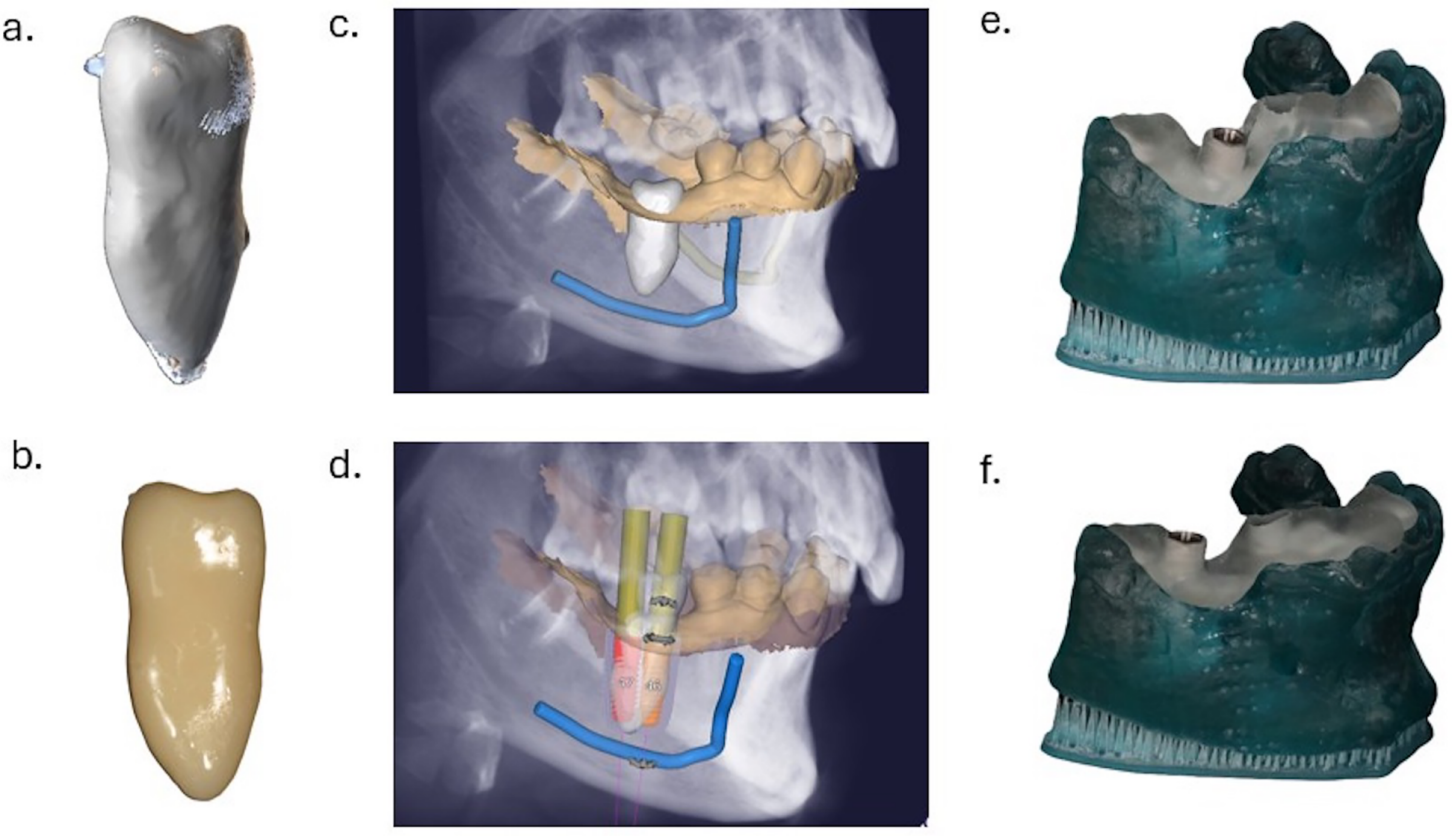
Digital planning for the autotransplantation procedure: digital segmentation of the donor tooth and the fabrication of a three-dimensional printed donor tooth replica; the digital file of the donor tooth was later placed digitally in the recipient site and two surgical guides following each of the donor tooth's roots were designed and printed. (a) Donor tooth segmentation from the CBCT file using BlueSkyPlan software. (b) 3D printed donor tooth replica. (c) Digital planning of the donor tooth position in the mandibular arch using Exocad software. (d) Planning osteotomy with two surgical guides for the neoalveolus. Since the donor tooth displayed two fused roots, two separate surgical guides were designed for each root. (e) Surgical guide for anterior root. (f) Surgical guide for posterior root.
